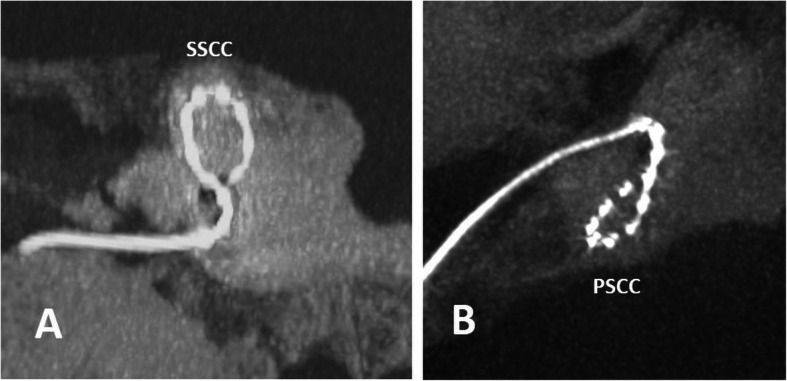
a A 86-year-old male patient, with immediate postoperative nausea. CBCT paracoronal maximum intensity projection image shows the malposition of the electrode in the superior semi-circular canal (SSCC). b A 20-year-old male patient with asymptomatic malposition of the electrode in the posterior semi-circular canal (PSCC) on CBCT paraaxial maximum intensity projection image
